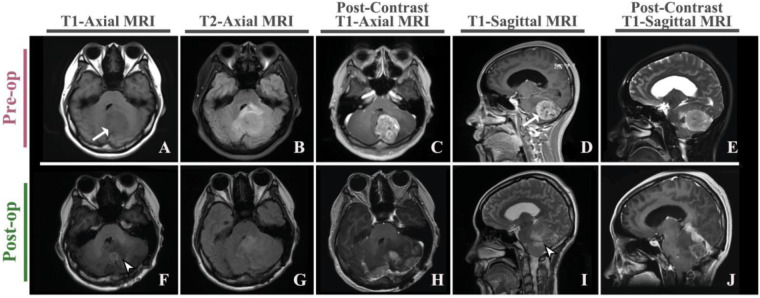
Pre- and postoperative radiological findings. (A) T1-weighted axial (preoperative); (B) T2-weighted axial (preoperative); (C) post-contrast T1-weighted axial (preoperative); (D) T1-weighted sagittal (preoperative); (E) post-contrast T1-weighted sagittal (preoperative). Arrows in preoperative panels indicate the left cerebellar hemispheric mass. (F–J) Corresponding postoperative sequences at matched planes demonstrating the resection cavity without definite residual enhancement; arrowheads indicate the postoperative cavity/resection bed (slice positions matched).
